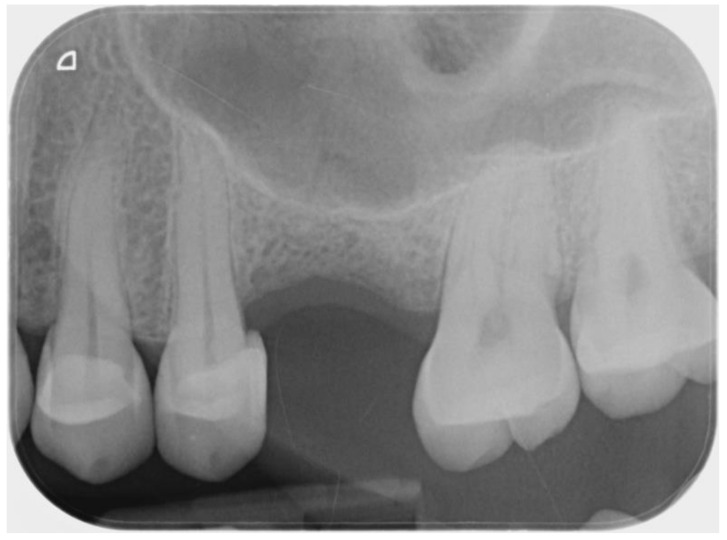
Intra-oral pre-operative x-ray of the edentulous site.
